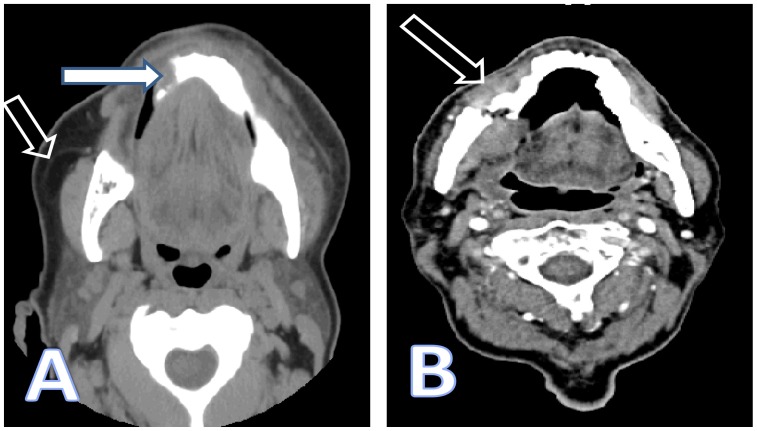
Computed tomography of the face without (A) and with (B) intravenous contrast. (A) Mandibular sclerosis with periosteal reaction of the mandibular body extending to the parasymphyseal region; given history of bisphosphonates therapy most likely osteonecrosis (full arrow). Diffuse subcutaneous edema and submental soft tissue swelling reflecting focal inflammatory changes (hollow arrow). (B) Osteoradionecrosis of the mandible with pathologic fracture of the right horizontal mandibular ramus (arrow).
